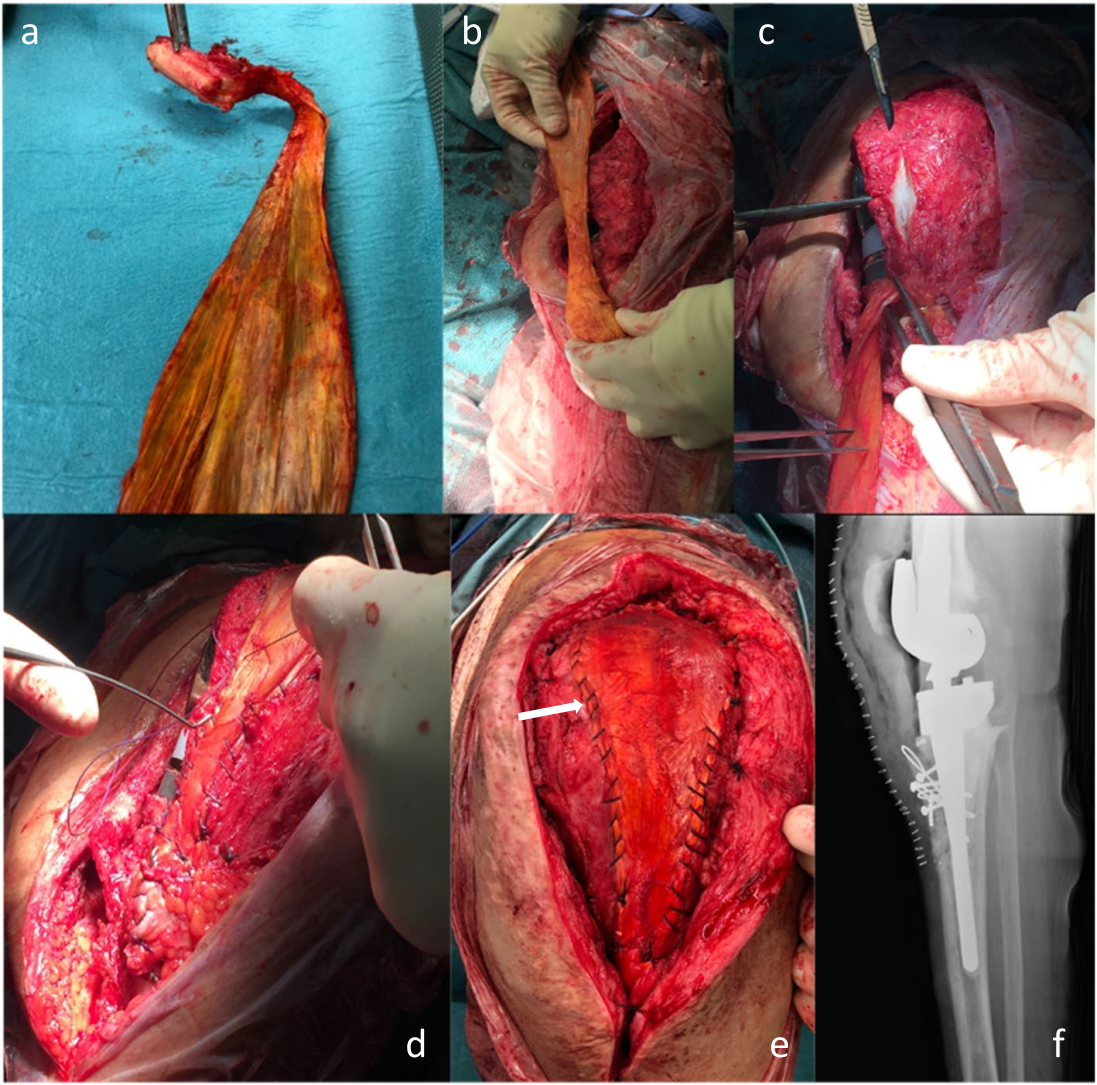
Different steps of extensor mechanism reconstruction. a: Achilles’ tendon allograft, b: graft preparation, c: patellar tendon split, d: the Achilles’ tendon was sutured with a number 2 nonabsorbable sutures in full extension, e: final placement of the graft, f: Radiographic evaluation
